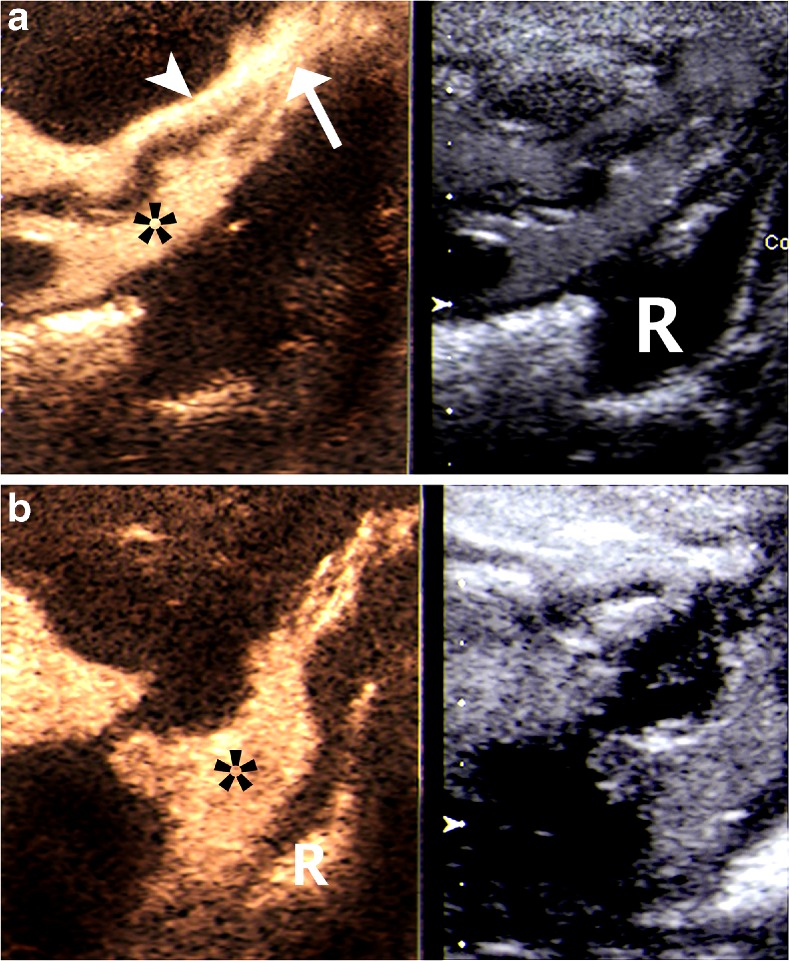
A newborn girl with cloacal malformation. Contrast-enhanced dynamic US genitography with perineal sagittal access is shown in a split dual image display with non-enhanced (left) and enhanced sonogram (right). a Contrast-filled urethra (*arrowhead*) and vagina (*asterisk*) with the respective common distal channel (*arrow*). The rectum is still filled with clear fluid (saline) from the earlier phases of the investigation (with saline instillation through the common external orifice). b At a later phase, there is US contrast agent both in the vagina (*asterisk*) and in the distal rectum (*R*)
